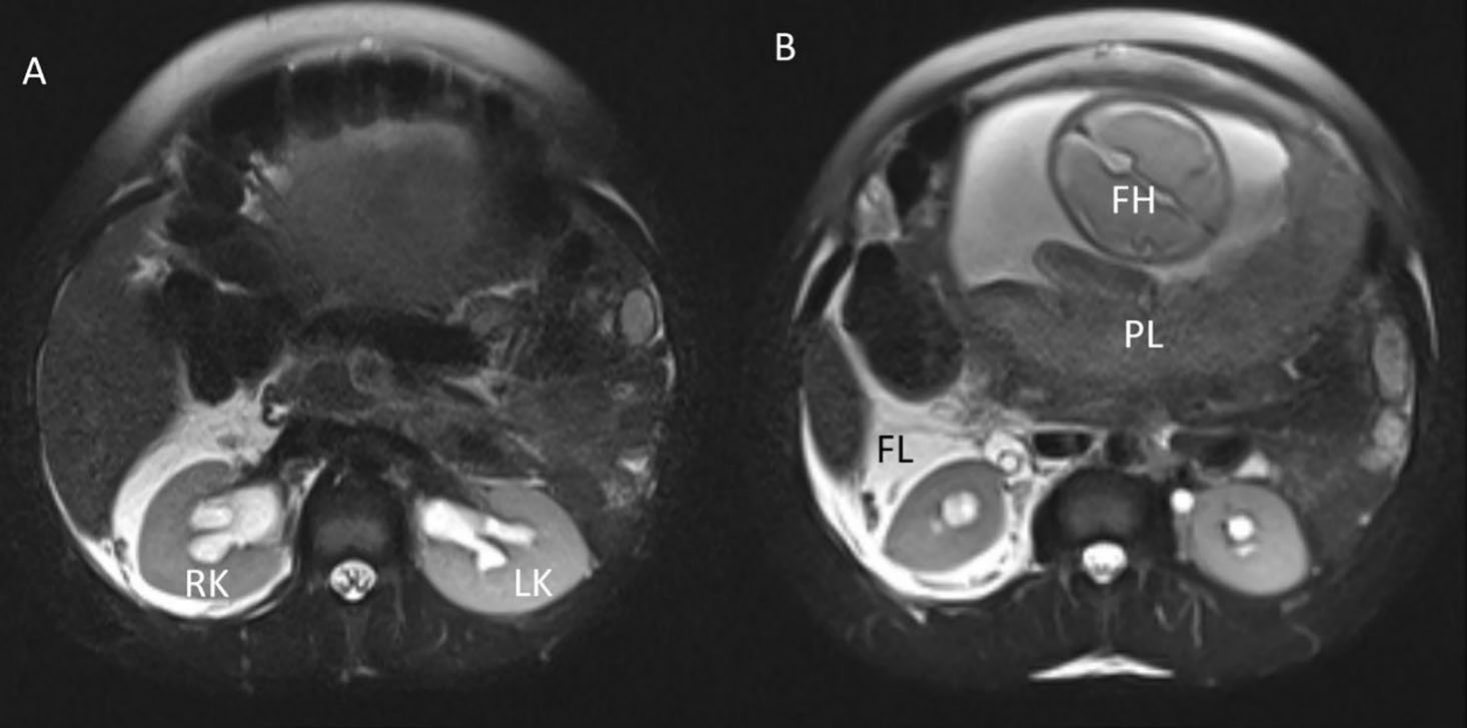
Axial T2W MRI images of the abdomen at the level of the kidneys. (A, B) show bilateral calyceal dilatation, with fluid (FL) around the right kidney (RK). (B) shows part of the gravid uterus with a fetal head (FH).
